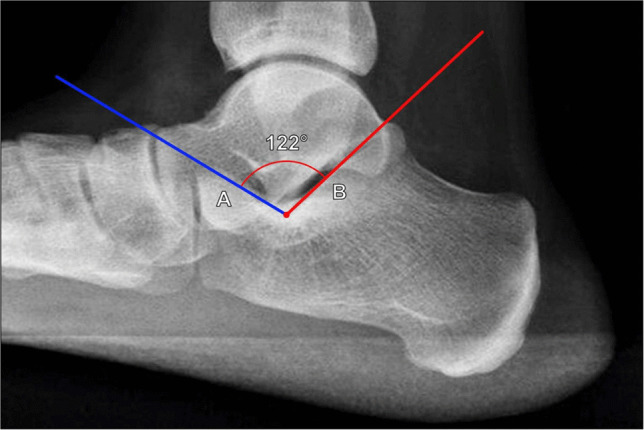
Measurement of the critical angle of Gissane on lateral weightbearing radiograph of the foot. The angle is formed by lines drawn along the superior surface of the anterior calcaneal process (A, blue line) and along the posterior calcaneal facet (B, red line). Generally accepted normal angles range from 120° to 145°. The critical angle of Gissane enlarges with greater posterior calcaneal facet collapse
